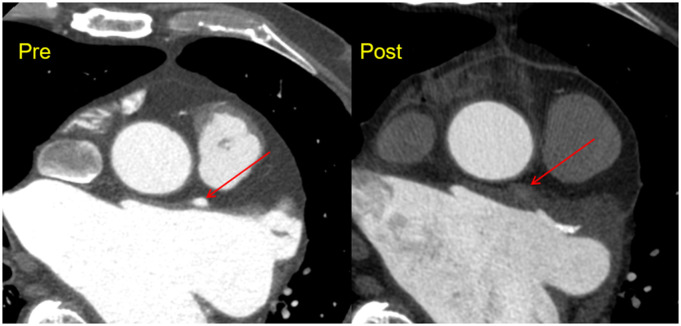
Postoperative Findings. Preoperative and postoperative CT images demonstrating elimination of the fistulous communication after hybrid treatment.
